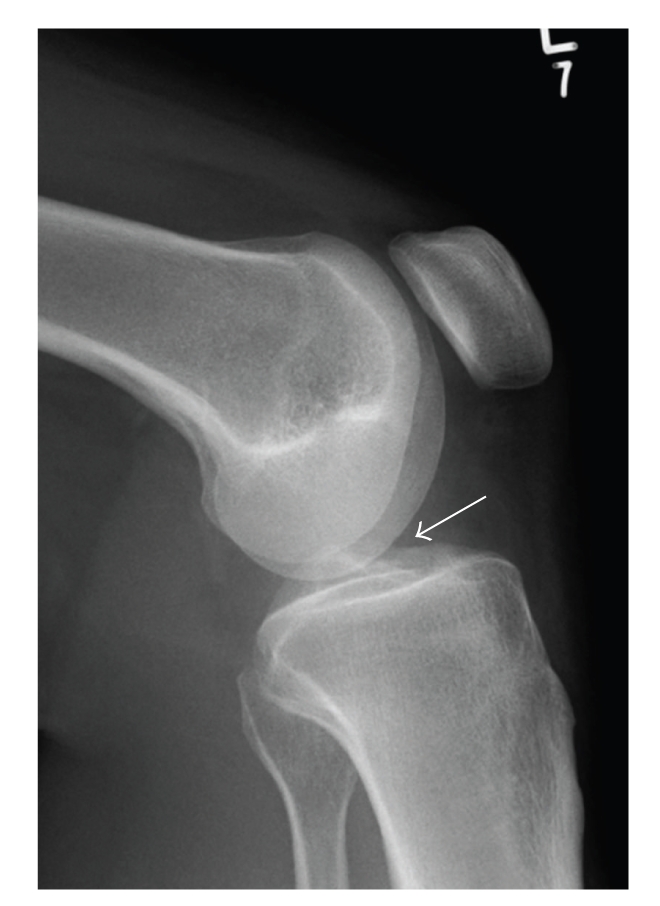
Lateral knee radiograph showing a soft tissue density lesion in the location of Hoffa's fat pad.
